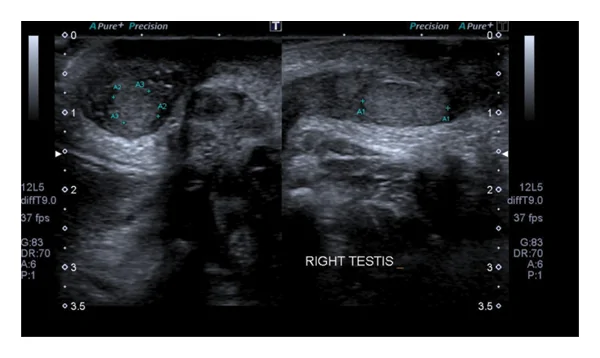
Postoperative ultrasound.
